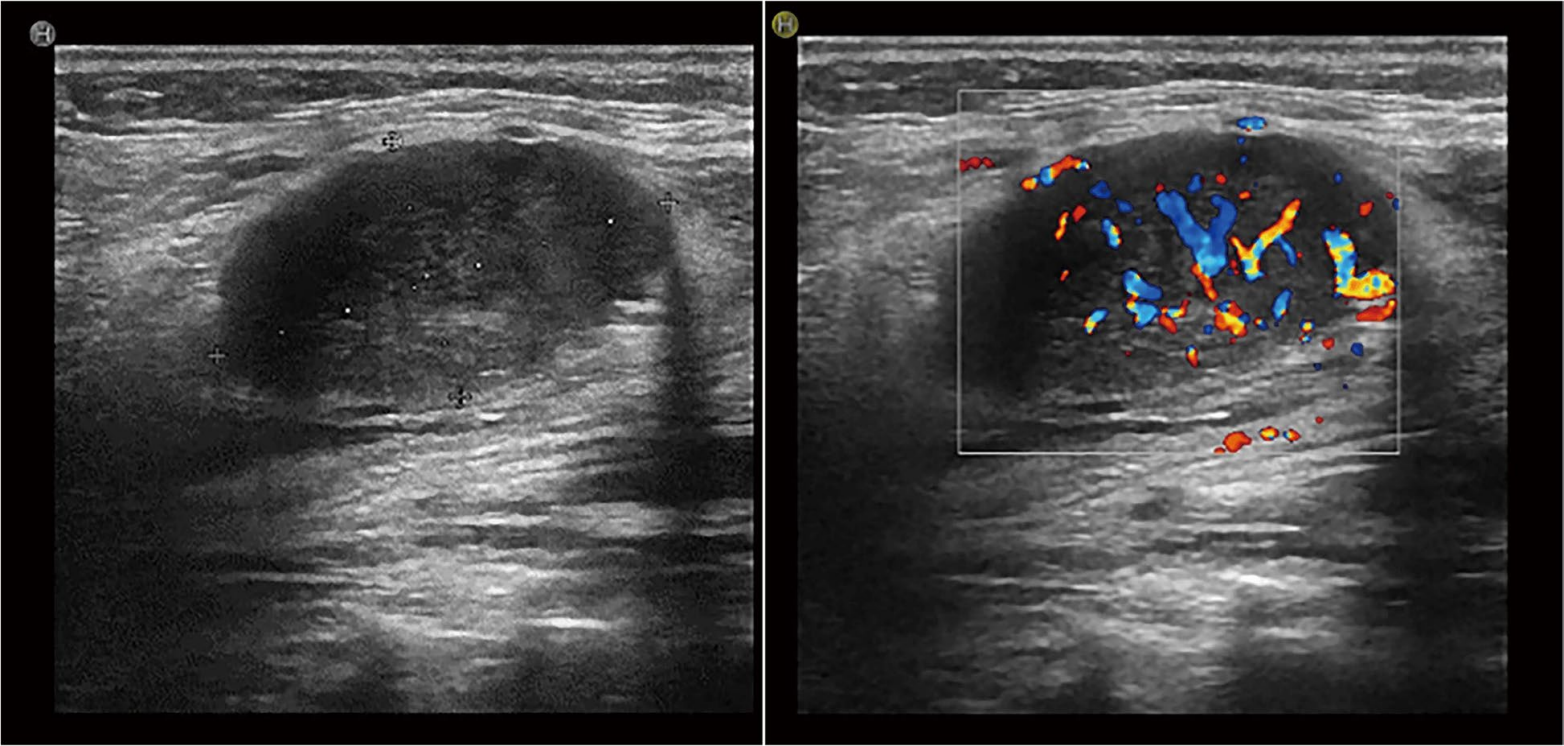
Ultrasound showed that a 2.2 cm × 1.7 cm nodule with blood flow signals
